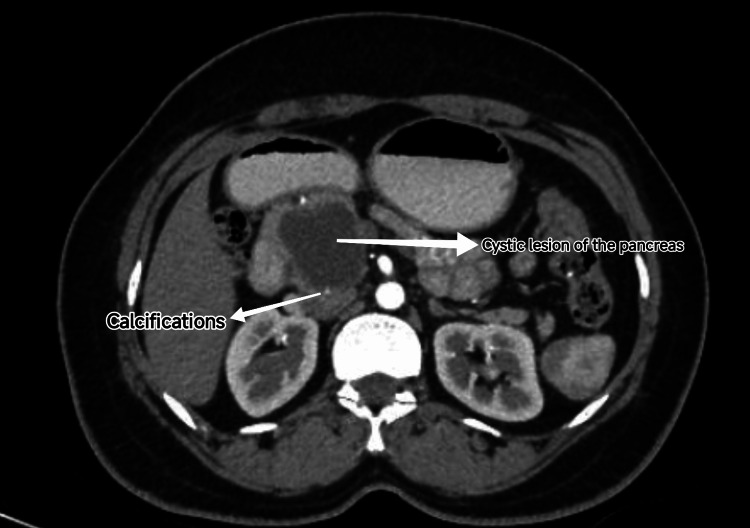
Contrast-enhanced CT abdomen Contrast-enhanced CT abdomen shows a thin-walled, multiloculated cystic lesion measuring 4 x 4 cm over the head and uncinate process of the pancreas, with peripheral septations and tiny calcifications. CT: computed tomography
